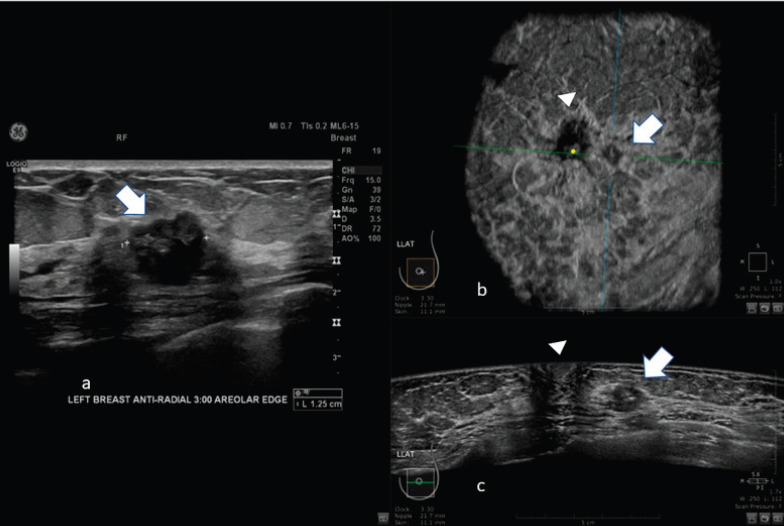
62-year-old patient with biopsy proven left breast carcinoma. a: Hand-held ultrasound showing the lesion (arrow). b: 3D ultrasound (ABUS) image at the dedicated workstation. Reconstructed coronal plane: the lesion is marked with the arrow; the nipple is marked with the arrowhead. c: reconstructed axial plane of Automatic Breast Ultrasound (ABUS): the lesion is marked with with the arrow; the nipple is marked with arrowhead.
